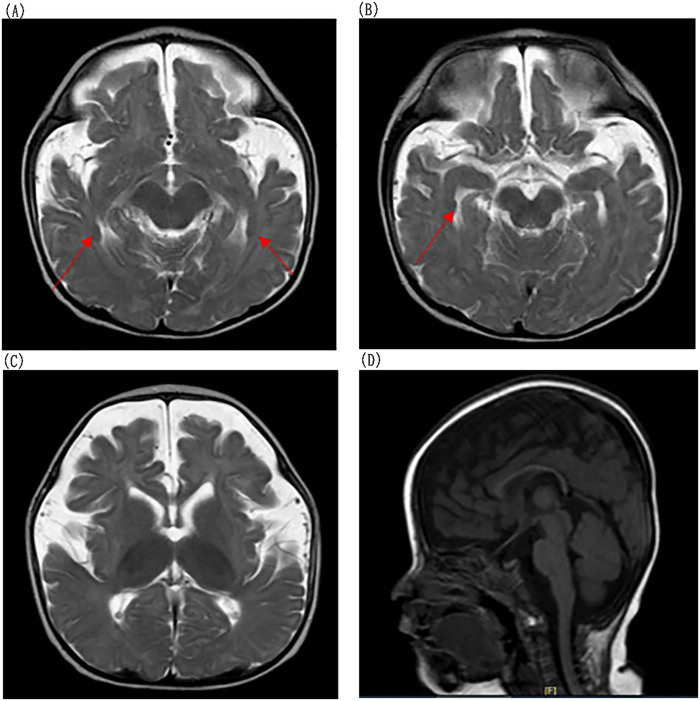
Brain MRI findings. (A,B) Periventricular nodular heterotopia (arrowheads) in patient; (C,D) Additional findings include small volume in the frontal and temporal lobes, prominent cerebral sulci and widened fissures, ventriculomegaly, and a thin corpus callosum.
